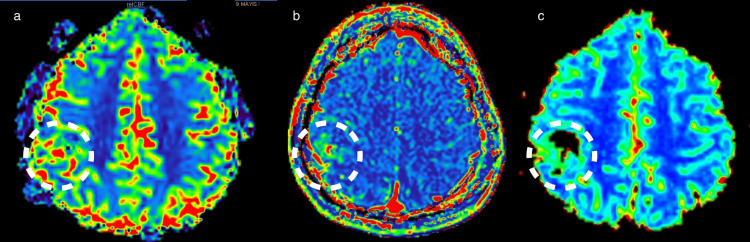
MRI T2 perfusion CBF, T1 perfusion fractional plasma volume and maximum relative enhancement images On the T2 perfusion CBF map (a), some parts of the lesion have elevated relative CBF values by up to four times. On T1 perfusion images, the lesion exhibits increased fractional plasma volume (b) and maximum relative enhancement values (c). CBF:  Cerebral blood flow.
